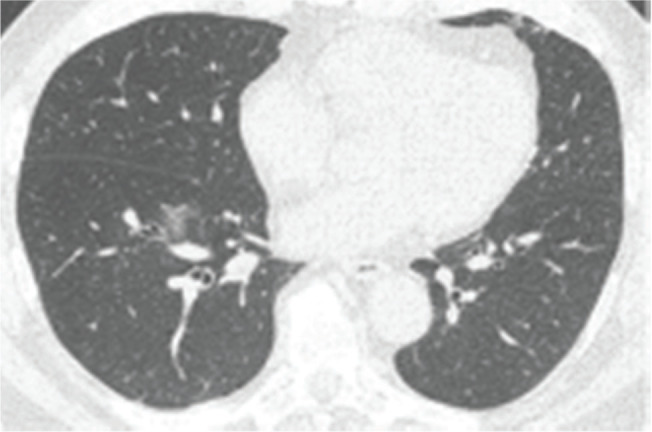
Indeterminate findings of COVID-19. Axial CT image shows a very small GGO with a non-rounded and non-peripheral distribution.
